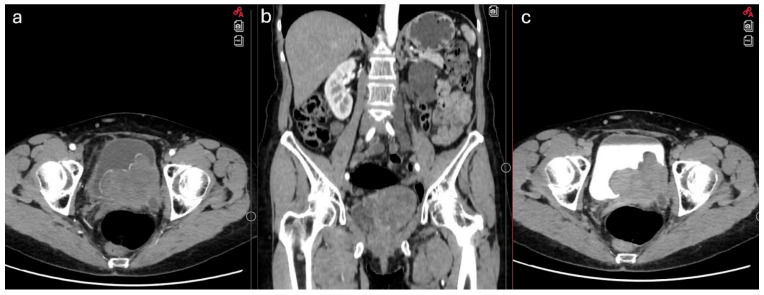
Preoperative CT showing a bladder mass. (a) axial view in the arterial phase; (b) coronal view; (c) axial view in the delayed phase.
